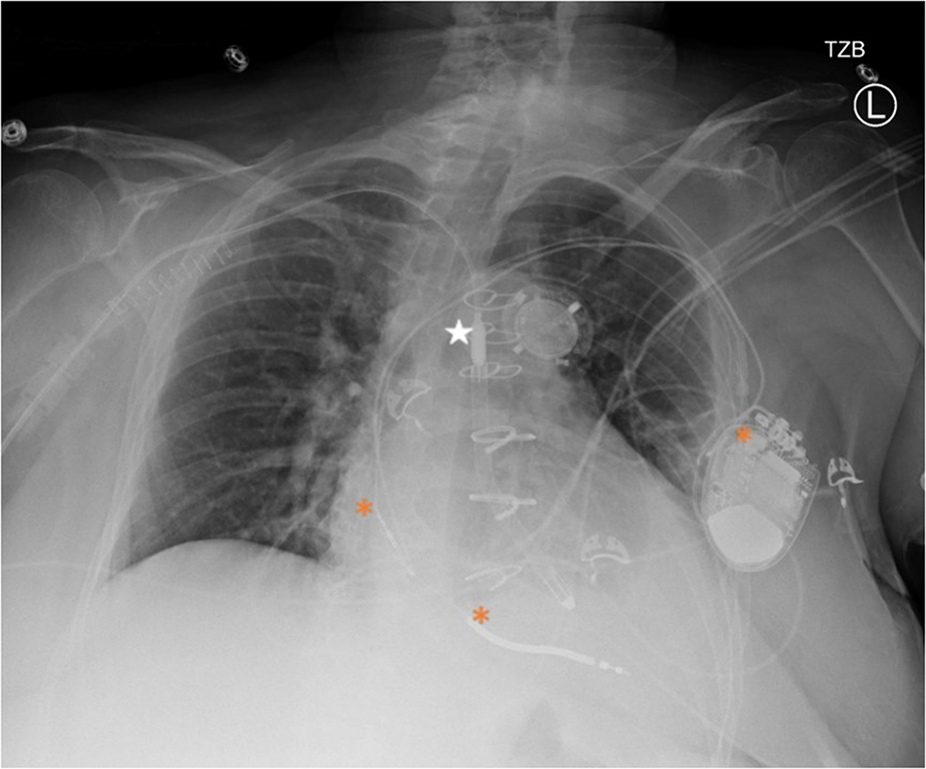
Portable chest x-ray after impella 5.5 placement. Star = Impella 5.5, * = ICD generator and leads in the right atrium and ventricle.
